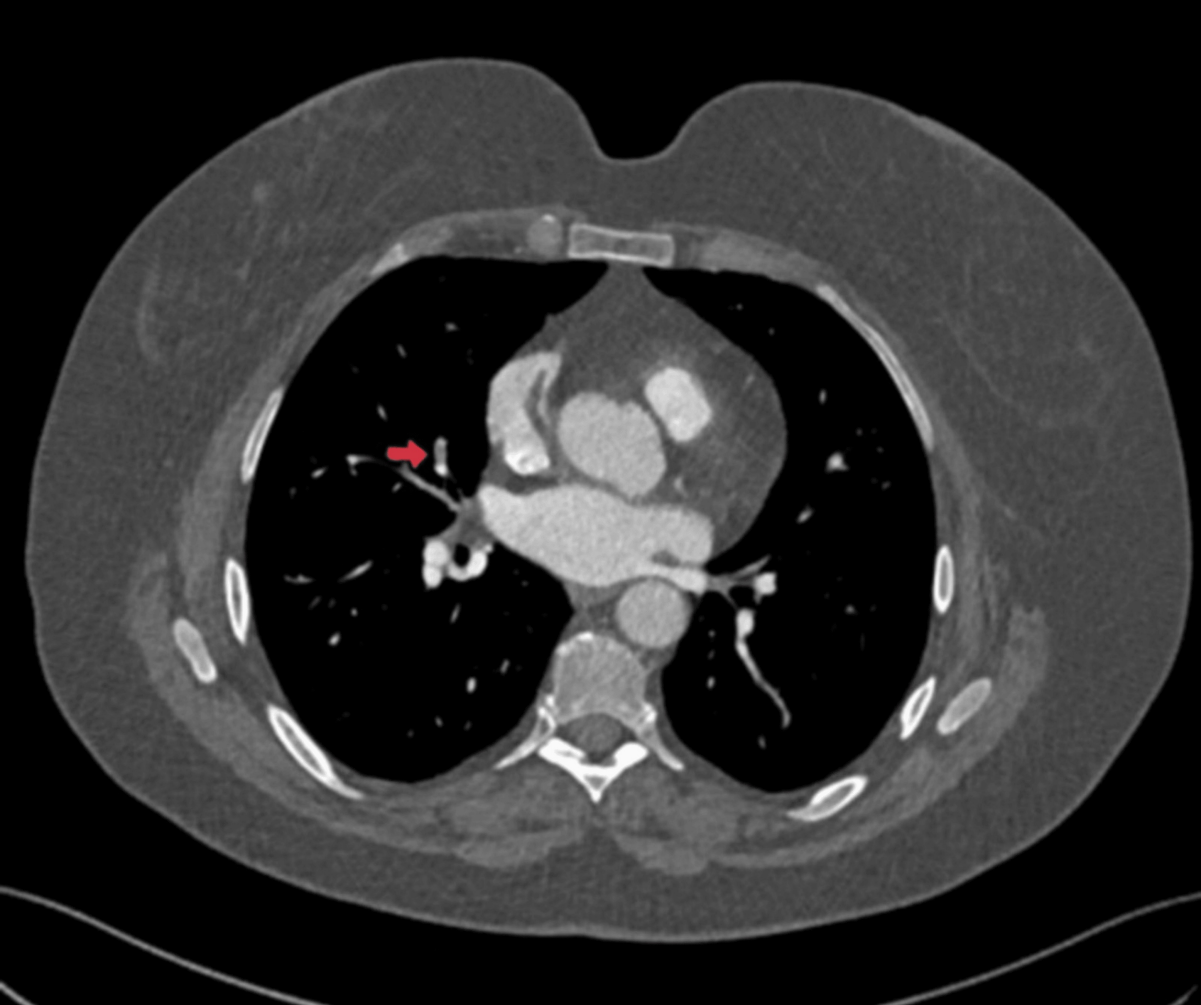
Axial CTPA image showing a pulmonary embolism in the middle lobe of the right lung (red arrow). CTPA: Computed Tomography Pulmonary Angiography.
